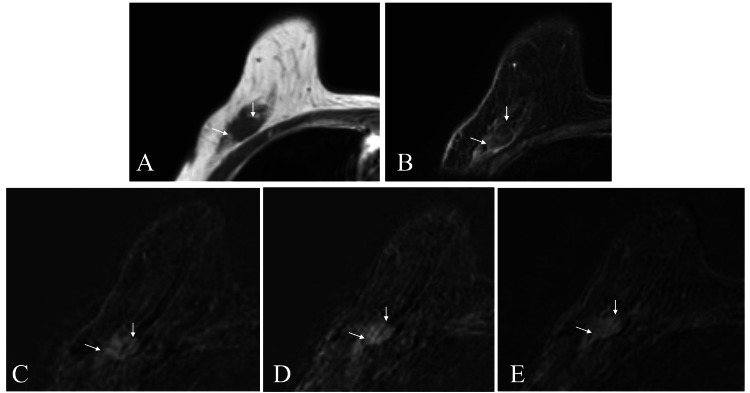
MRI findings The tumor showed a hypo-intense pattern on T1- and fat-suppressed T2-weighted images (A and B). Time-signal intensity images (C: one minute, D: two minutes, and E: six minutes) showed a persistent pattern. MRI: magnetic resonance imaging
